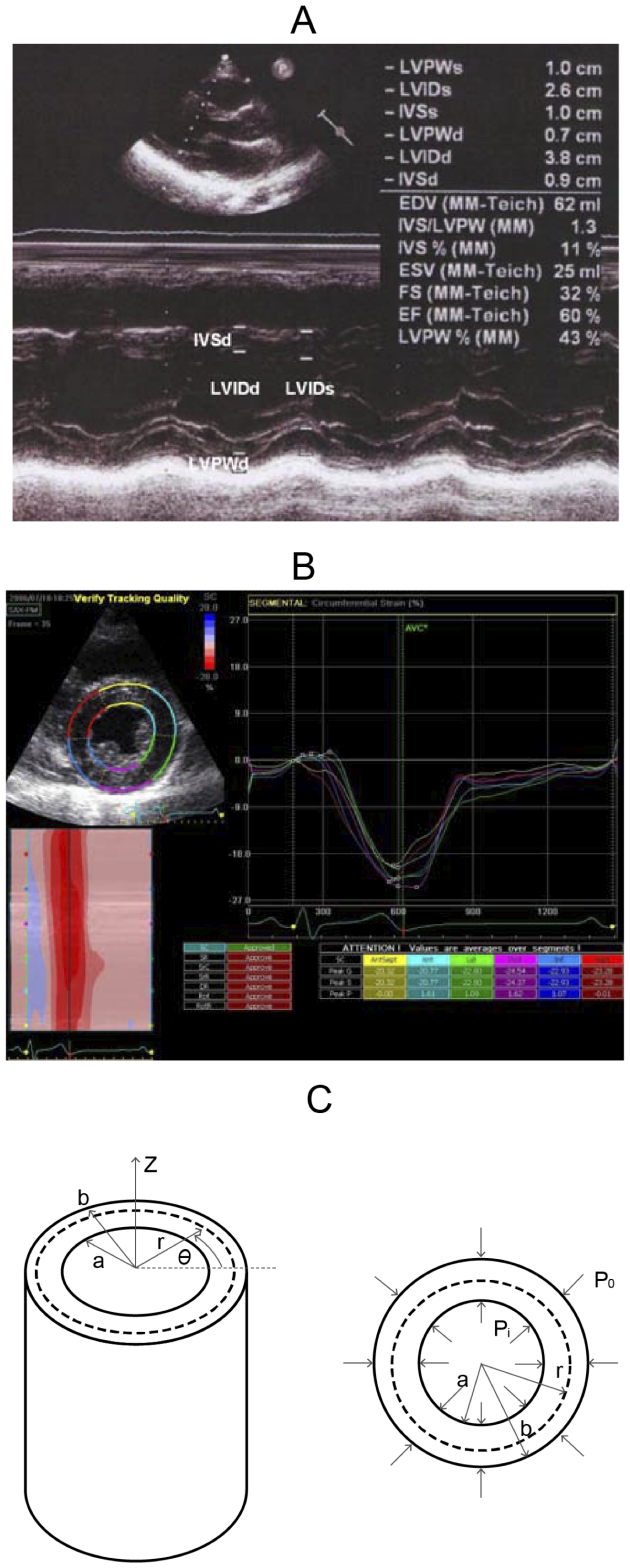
The echocardiographic parameters and the mathematical model for computing the myocardium stiffness of the left ventricle. A: The measured echocardiographic parameters. IVSd: intraventricular septal width in diastole; LVIDd: left ventricular internal dimension in diastole; LVPWd: left ventricular posterior wall width in diastole; and LVIDs: left ventricular internal dimension in systole. B: The measuring circumferential strain in the short-axis, at the papillary muscle level. C: The model of the cylinder, gross and cross-section.
